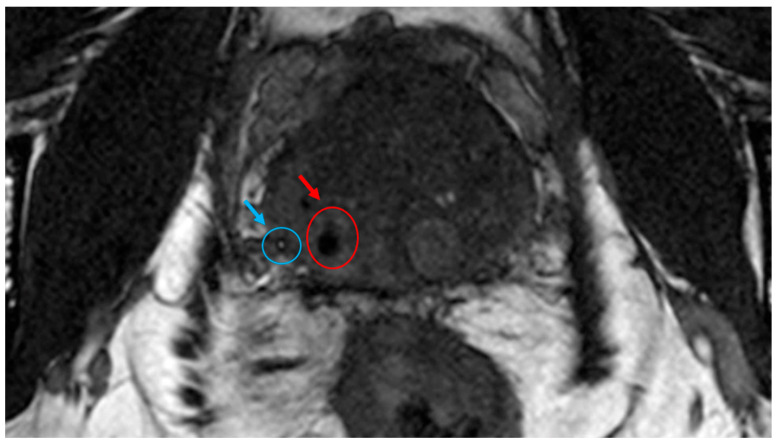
Visibility of NOVA and Gold Anchor fiducial markers on an MR image of a patient undergoing proton therapy for prostate cancer. NOVA markers (blue circle and blue arrow) are visualized as a positive signal, whereas Gold Anchor markers (red circle and red arrow) presented as a susceptibility artifact.
